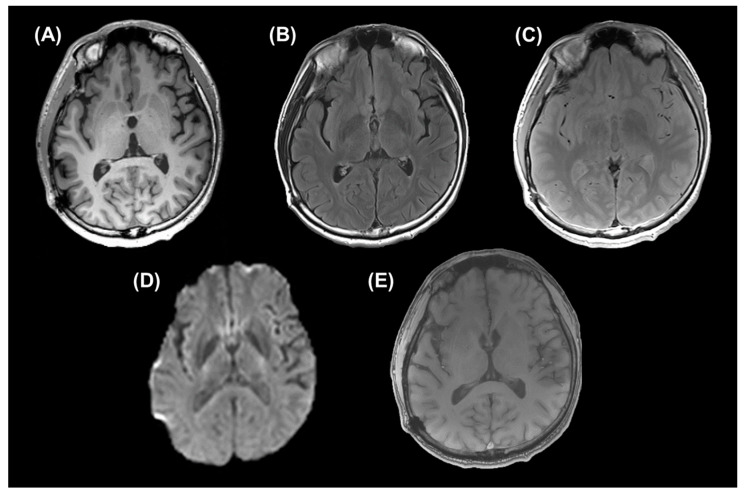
Conventional MRI sequences of a patient {48-year-old male with mild traumatic brain injury (TBI) in chronic phase}. (A) An axial reconstructed image of magnetization-prepared rapid acquisition gradient-echo (MPRAGE) sequence (repetition time (TR)/ echo time (TE)/ inversion time (TI) = 1900/2.85/900 ms), (B) an axial fluid-attenuated inversion recovery (FLAIR) image (TR/TE/TI = 12,000/115/2800 ms), (C) an axial proton density-weighted image (PDWI) (TR/TE = 4000/12 ms), (D) trace of an axial diffusion imaging sequence (TR/TE = 4000/83.2 ms, maximum b-value = 8000 s/mm^2^), and (E) an axial 3D gradient-echo susceptibility-weighted image (SWI) (TR/TE = 24/3.9 ms, flip angle (FA) = 73°) are given.
